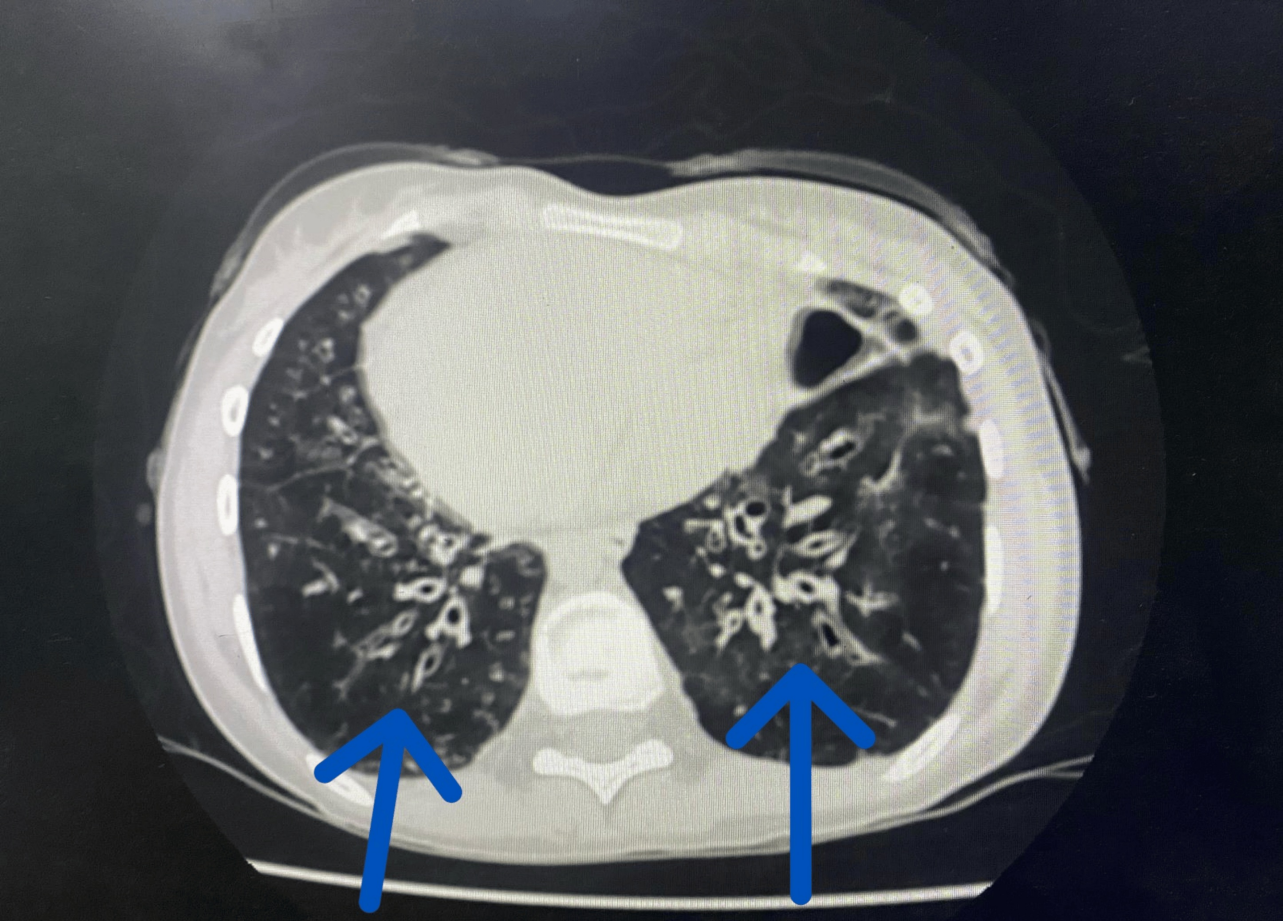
High-resolution CT scan of the chest shows the characteristic 'signet ring' sign, indicative of bronchiectasis.
